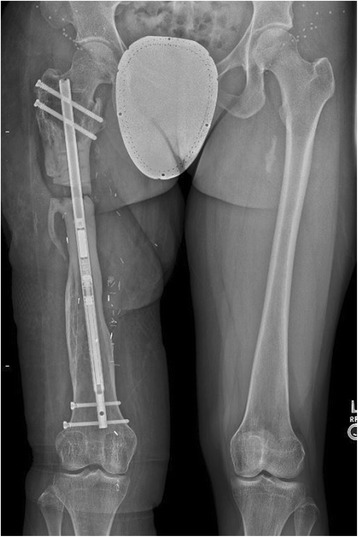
Long leg weight-bearing plain films demonstrating femur after one week of lengthening
